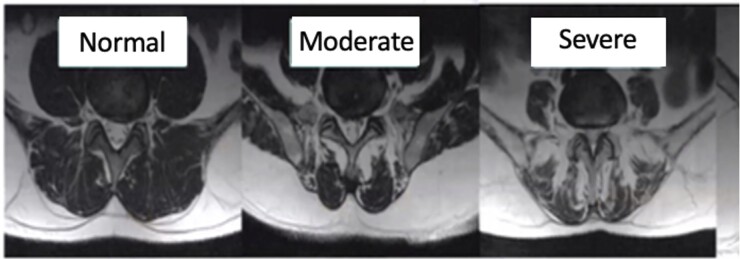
Magnetic resonance images demonstrating progressive multifidus fat infiltration from normal to moderate to severe.
